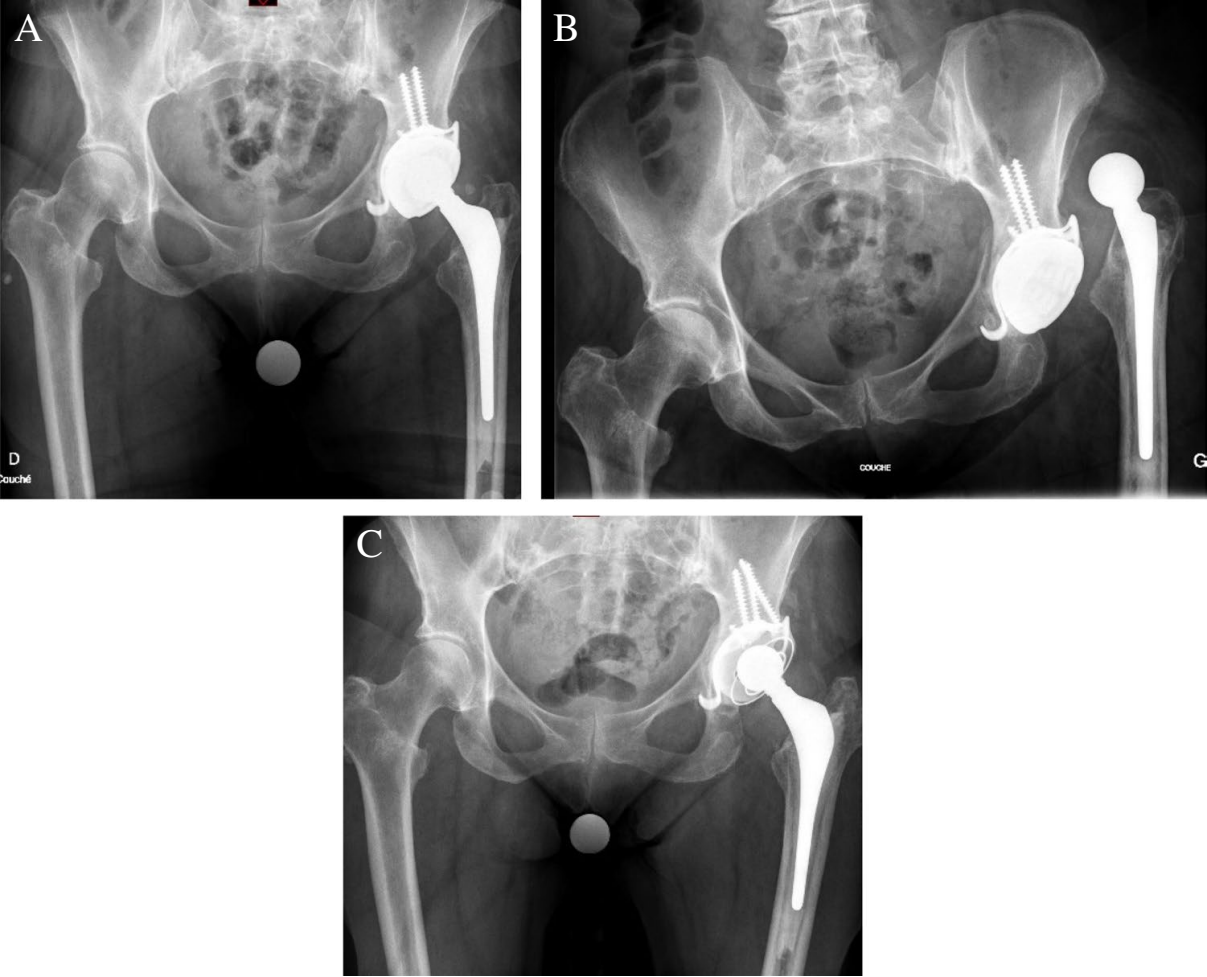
Case of a 72-year-old female patient presenting recurrent instability and required revision to a constrained liner. A Postoperative antero-posterior radiograph of the pelvic with revision using cemented dual mobility. B At 2 weeks, first dislocation episode. C After re-revision using a cemented constrained liner for persistent instability
